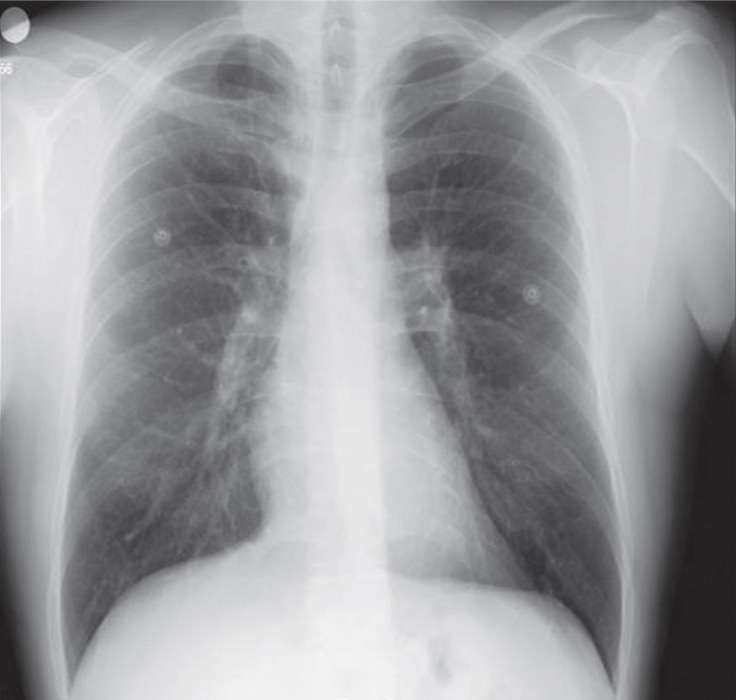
Chest X-ray, showing no accessory cervical rib
